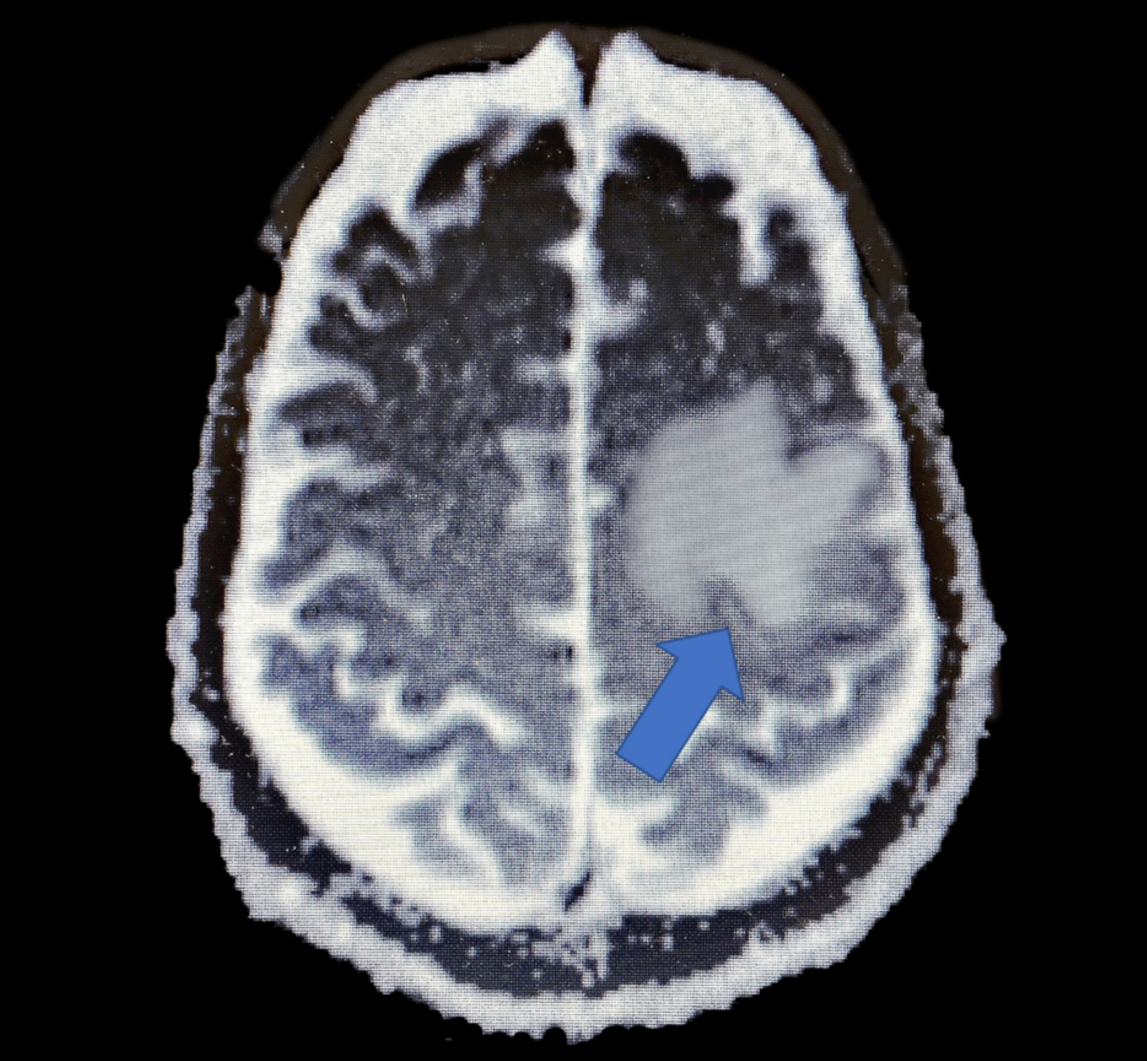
Pre-craniotomy MRI showing an enhancing mass with associated vasogenic edema in the posterior left frontal lobe consistent with brain metastasis.
